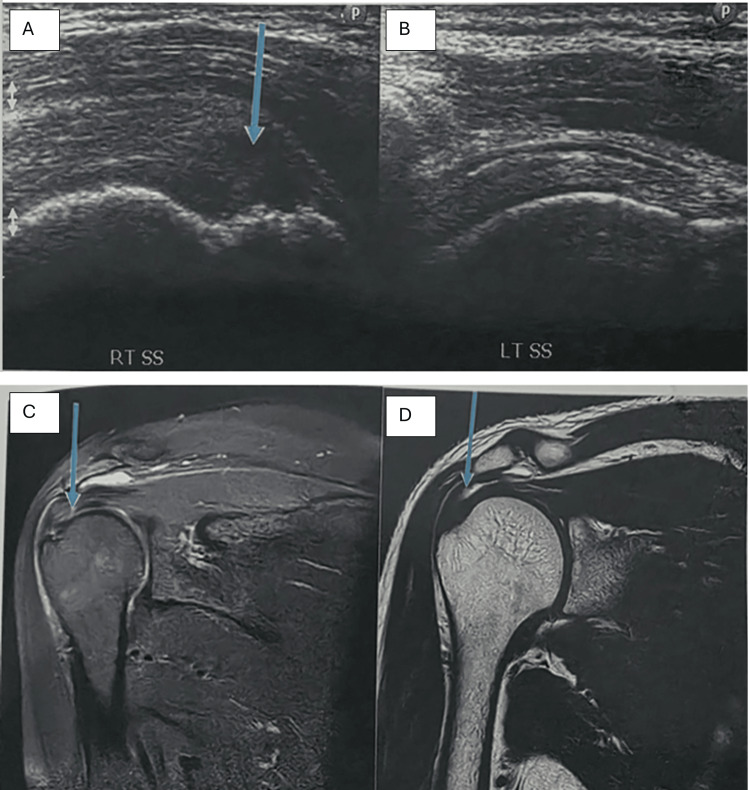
Partial-thickness supraspinatus tendon tear. (A-B) USG showing hypoechoic thickening in the supraspinatus tendon near its insertion over the greater tuberosity (arrow). (C-D) PD-weighted and T2-weighted MRI confirming a partial-thickness tear at the supraspinatus tendon insertion USG, ultrasonography; MRI, magnetic resonance imaging; PD, proton density
